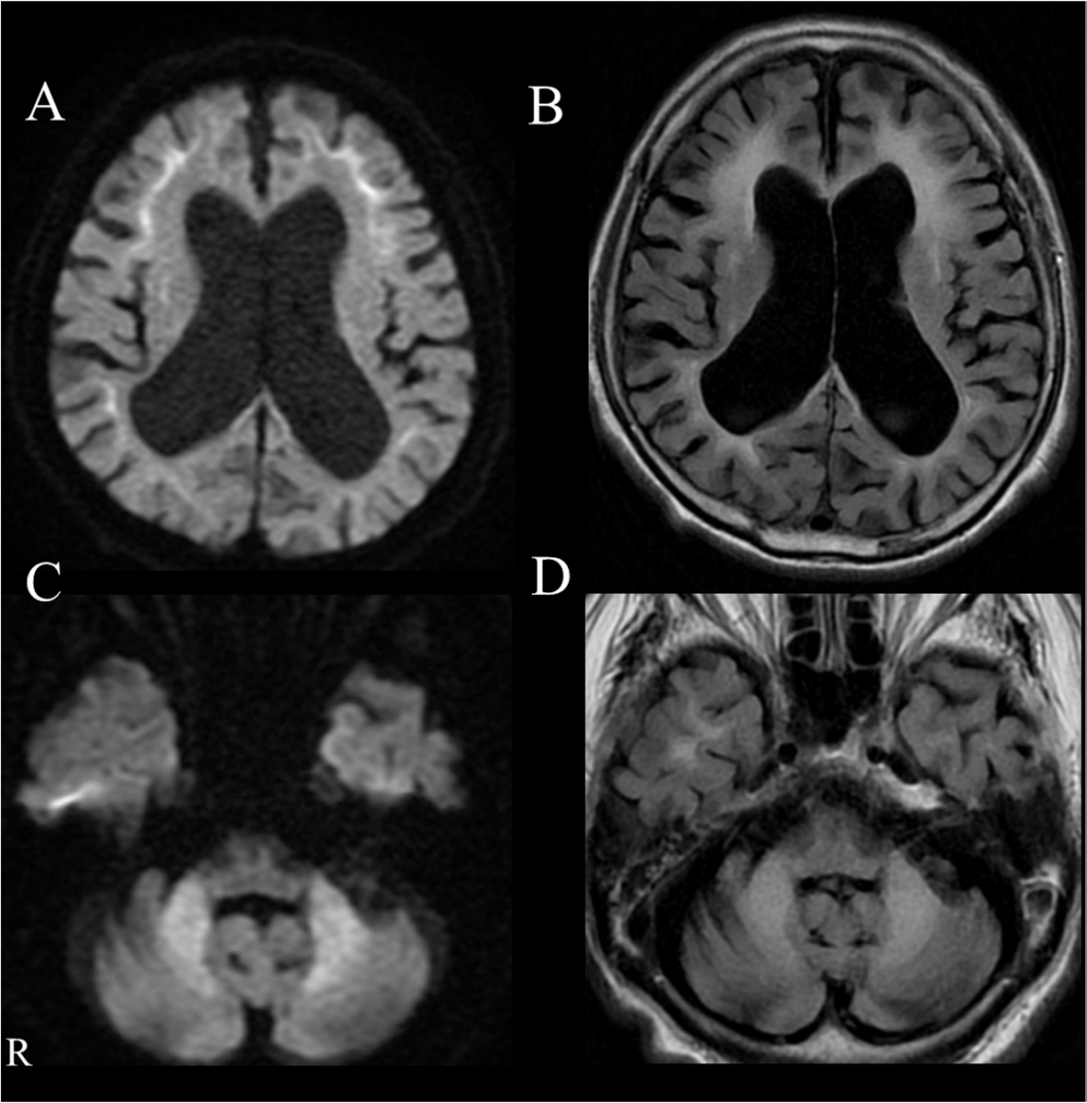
A–D Head MRI findings; an axial DWI showing high signal intensity along the corticomedullary junction (A). An axial FLAIR image showing diffuse atrophy of the cerebrum and increased signal intensity in the cerebral white matter (B). Bilateral abnormal high signal intensity in the middle cerebellar peduncles (MCP sign) is shown on axial DWI (C) and FLAIR (D)
